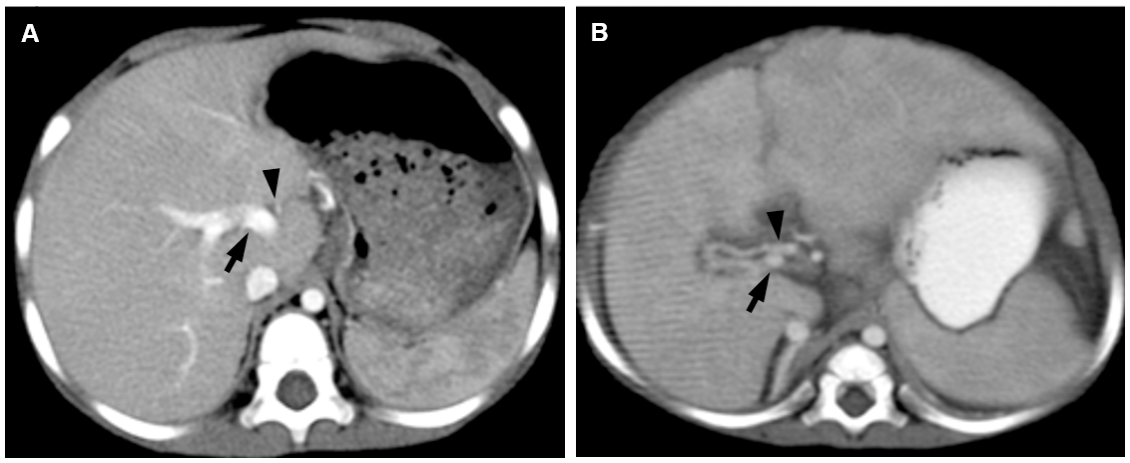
Contrast-enhanced axial CT scans for vascular changes. (A) Normal hepatic vasculature in a 5-month-old boy with Alagille syndrome. Diameters of proximal right hepatic artery (arrowhead) and portal vein (thin arrow) are 1.3 mm and 5.0 mm, respectively. The ratio of the hepatic artery diameter to the portal vein diameter is 0.26. (B) Enlarged hepatic artery and small portal vein diameters in a 6-month-old girl with biliary atresia. Diameters of proximal right hepatic artery (arrowhead) and portal vein (thin arrow) are 2.4 mm and 2.6 mm, respectively. The ratio of the hepatic artery diameter to the portal vein diameter is 0.92.
